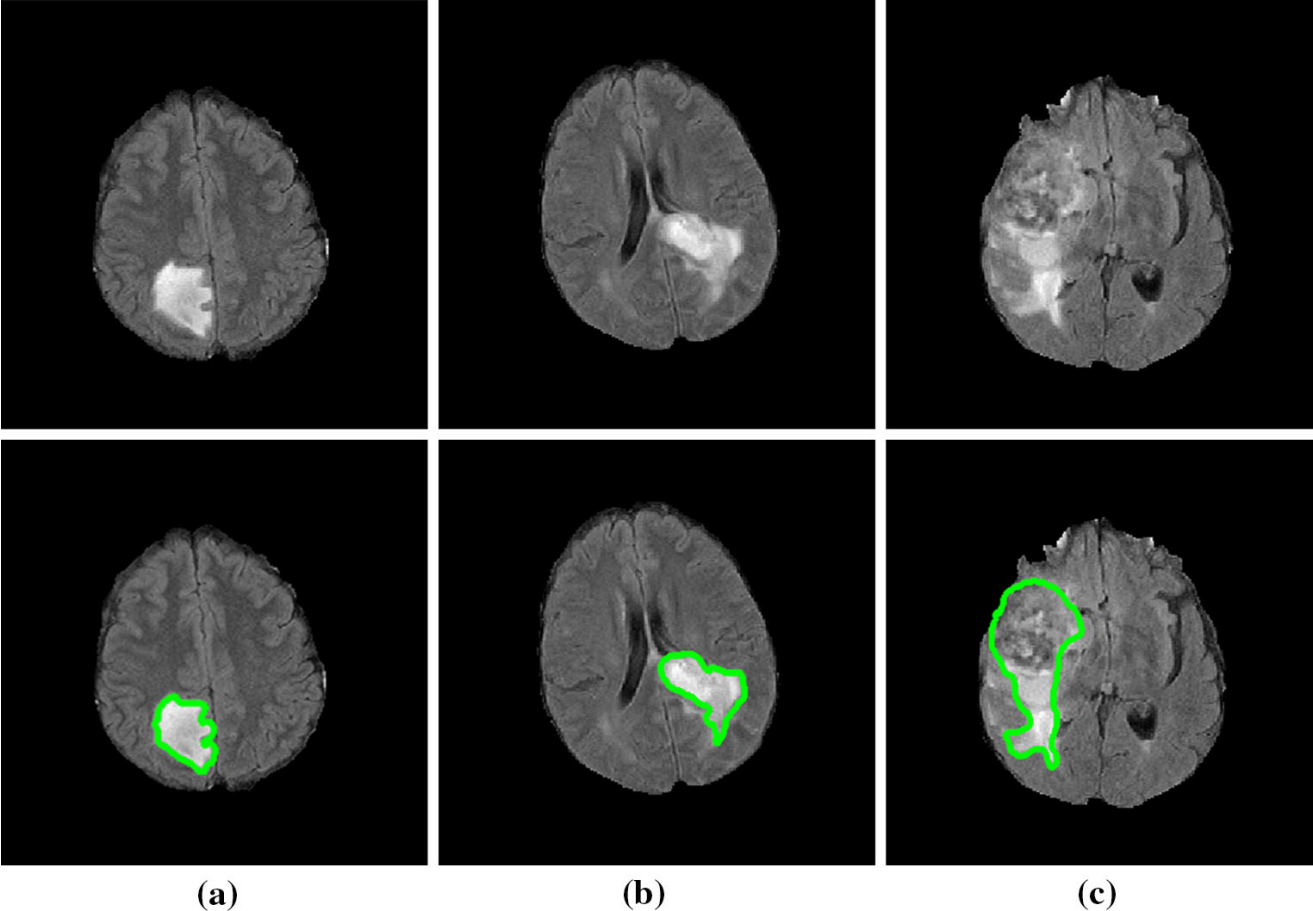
FLAIR images with different tumour grades in *upper row* and their ground-truth manual segmentation of the FLAIR hyperintensity in the *lower row*. Tumour grades are: a grade II, b grade III and c grade IV
